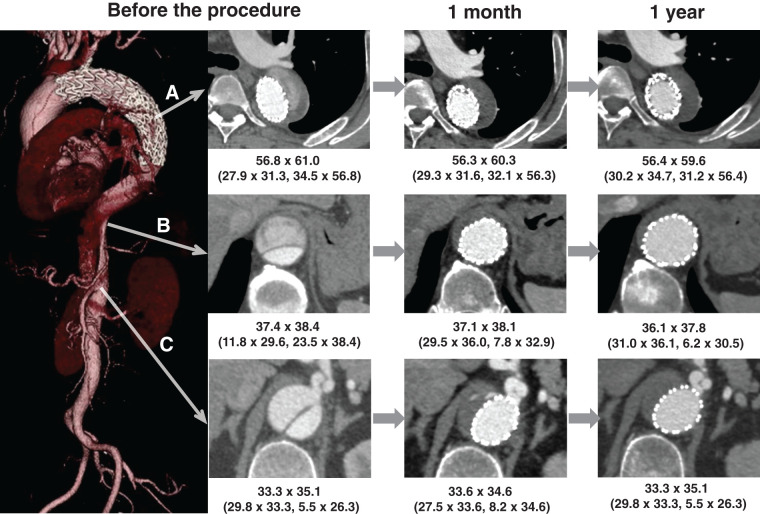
CT images of case 1 at 3 time points: preoperatively, 1 month, and 1 year after the STABILISE procedure. Aortic diameter (short axis × long axis, mm); TL and FL diameters are shown in parentheses. (A) Descending thoracic aorta at the level of the tracheal bifurcation. Preoperatively, FL expansion was observed due to retrograde flow; this resolved at 1 month. At 1 year, FL was thrombosed with aneurysm shrinkage. (B) Descending thoracic aorta at the level of thoracic vertebrae (T) 7 to 9. Preoperatively, a patent FL was present. At 1 month, TL was dilated and FL was obliterated. At 1 year, FL continued to be obliterated. (C) Abdominal aorta at the level of the celiac artery bifurcation. Preoperatively, the celiac artery originated from both TL and FL. At 1 month, FL thrombosed while the celiac artery perfusion from TL persisted. At 1 year, FL remained thrombosed. FL: false lumen; STABILISE: stent-assisted balloon-induced intimal disruption and relamination in aortic dissection repair; TL: true lumen; CT: computed tomography
